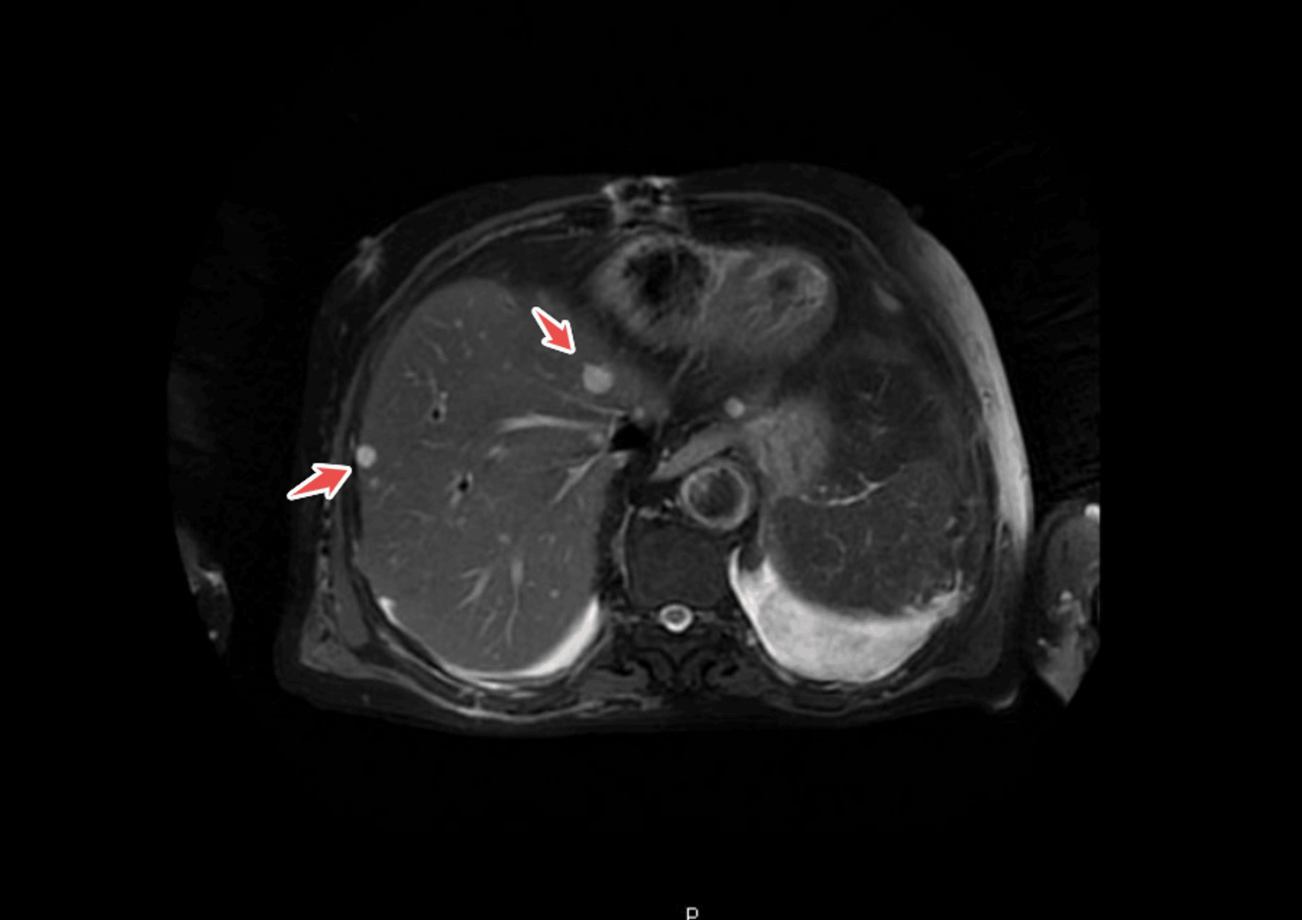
Axial abdominal MRI demonstrates multiple focal hepatic lesions (highlighted by arrows) MRI, magnetic resonance imaging
